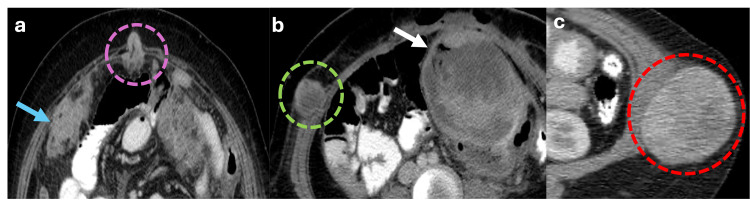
Axial abdominal CT scan with intravenous contrast showing a Sister Mary Joseph nodule, abdominal wall metastasis, and abdominal wall sarcoma (a) Axial CT scan shows a soft-tissue lesion with irregular borders around the umbilical region, also known as the sister Mary Joseph nodule (pink dotted circle), in a patient with advanced peritoneal carcinomatosis due to ovarian cancer (blue arrow). (b) Axial CT scan with intravenous contrast from another patient shows an irregular solid abdominal wall nodule with a necrotic core (green dotted circle) consistent with a metastatic deposit in a patient with a gastrointestinal stromal tumor (GIST) (white arrow). (c) Axial CT scan with intravenous contrast reveals a large oval-shaped mass with smooth borders and heterogeneous contrast enhancement located in the left lateral abdominal wall, partially invading the intramuscular structures, these findings in the context of a patient with no other neoplastic lesions is highly suggestive of a primary sarcoma (red dotted circle). The figure was created by the authors.
